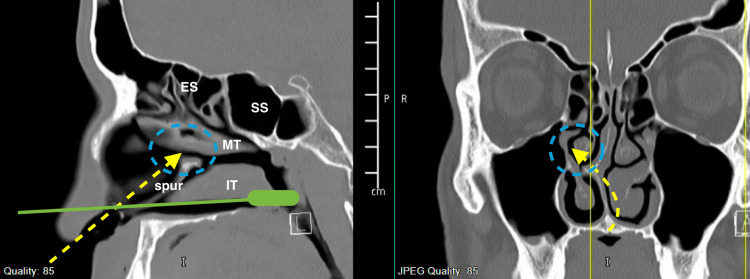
CT scan sinus. (Left) Sagittal cut. (Right) Coronal cut. CT: computed tomography; ES: ethmoid sinus; SS: sphenoid sinus; IT: inferior turbinate; MT: middle turbinate. Blue dotted circle: area of potential damage from the nasopharyngeal swab. Yellow dotted arrow: the improper trajectory of the nasopharyngeal swab. Green solid-line swab demonstrating the proper direction of nasopharyngeal swab.
